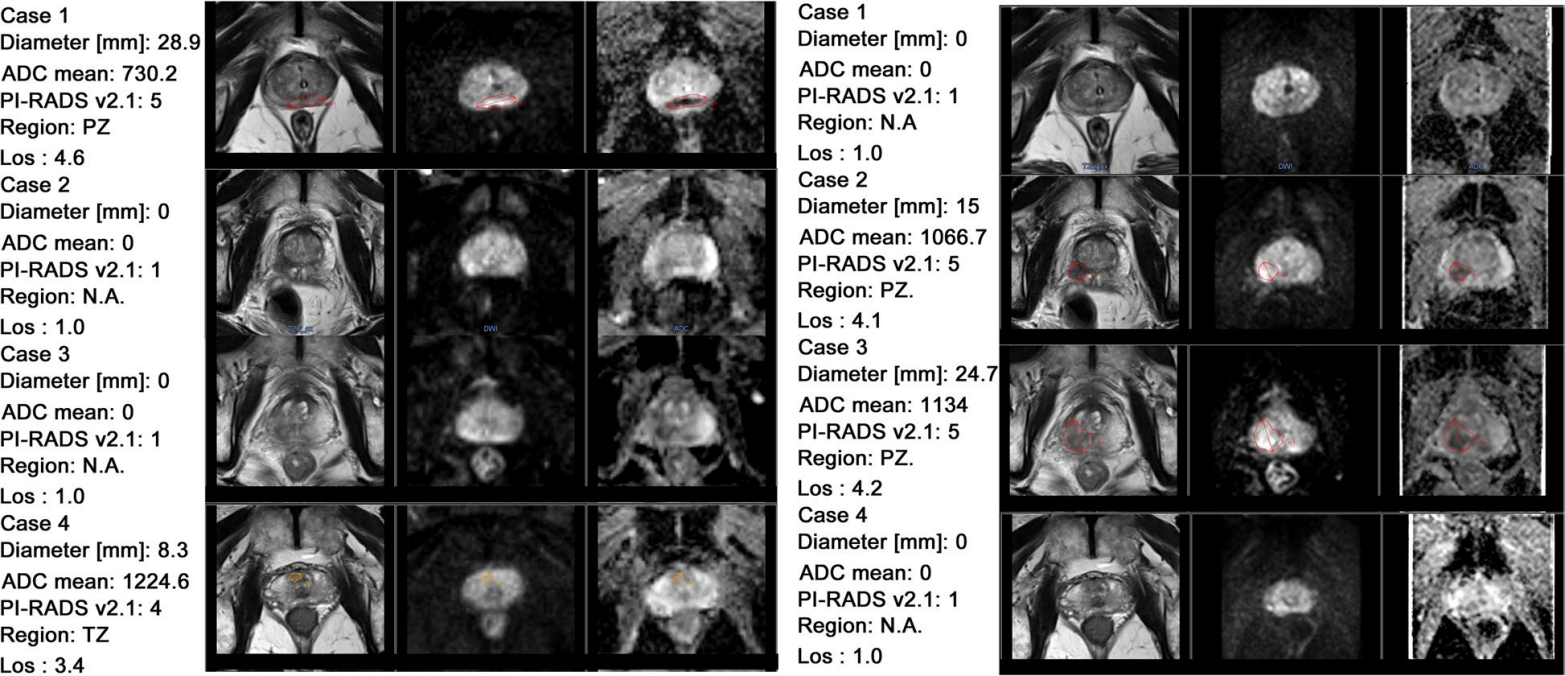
Examples of DL-CAD diagnosis using f-DWI (a) and z-DWI (b). The red or orange ROIs indicate highly suspected PCa lesions detected by the system. Cases without ROIs indicate no suspected PCa lesion was detected. Case 1. A 77-year-old man with an inflammatory lesion in the central zone (CZ). DL-CAD using f-DWI detected a fake cancer lesion in PZ, which was a rectal artifact. Case 2. A 62-year-old man with a PCa lesion (Gs = 3 + 4) in the right PZ, which was missed by DL-CAD with the use of f-DWI possibly due to rectal distortion and artifacts. Case 3. A 71-year-old man with an inflammatory lesion in the right PZ, which was detected by DL-CAD using z-DWI. Case 4. A 73-year-old man with a PCa lesion (Gs = 4 + 3) in the transition zone (TZ) which was missed by DL-CAD using z-DWI
